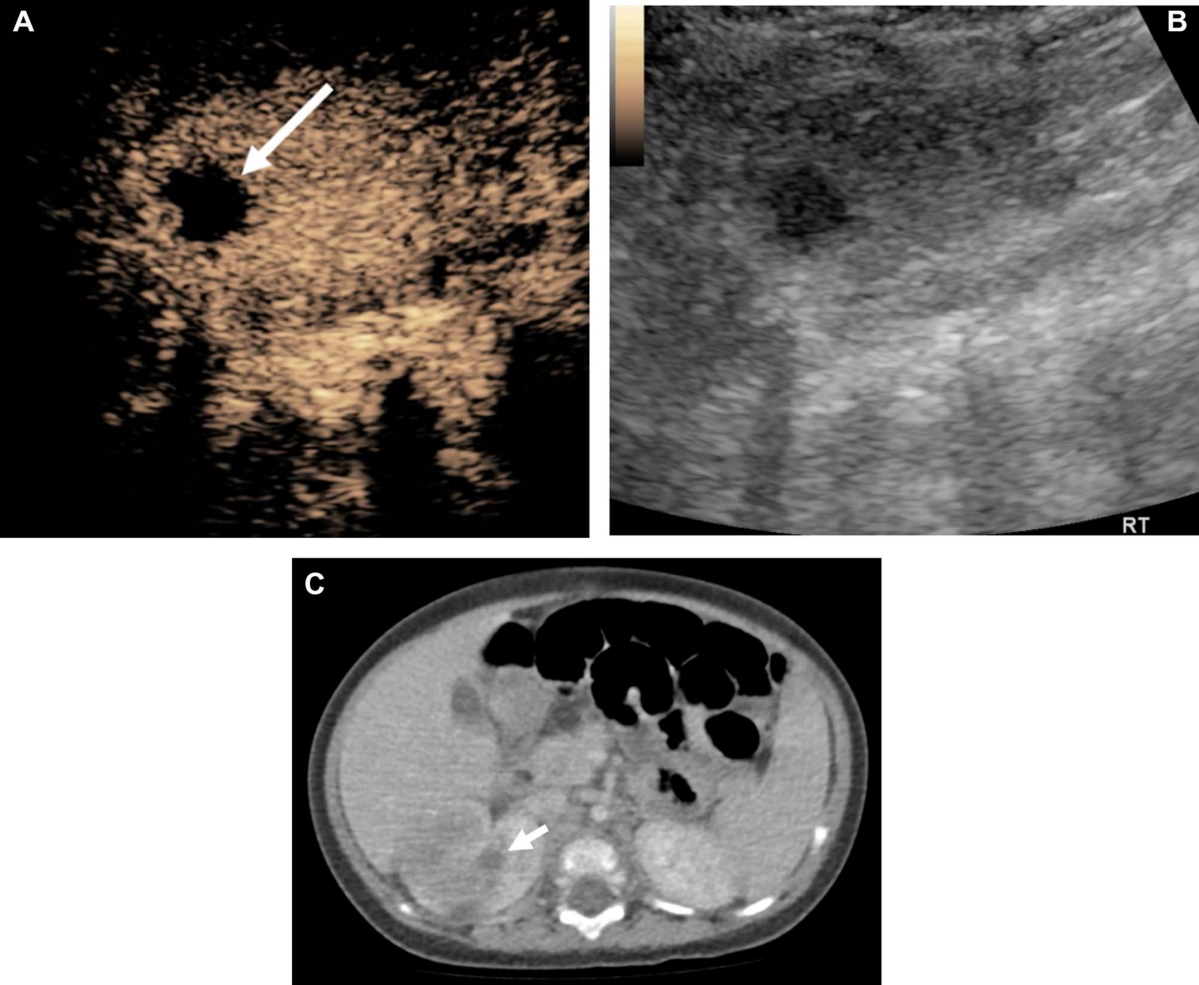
A 5-month-old male with acute pyelonephritis. The CEUS scan on the axial plane (A) with the gray-scale image (B) shows a hypoechoic lesion at the right kidney cortex (arrow) within the larger area of the hypoenhancing renal parenchyma. The lesion was not enhanced during the enhancement period and interpreted as an abscess. The CT image (C) shows a round-shaped non-enhancing lesion (correlating with the abscess on CEUS) (arrow) within a larger area of hypoenhancement (acute pyelonephritis).
